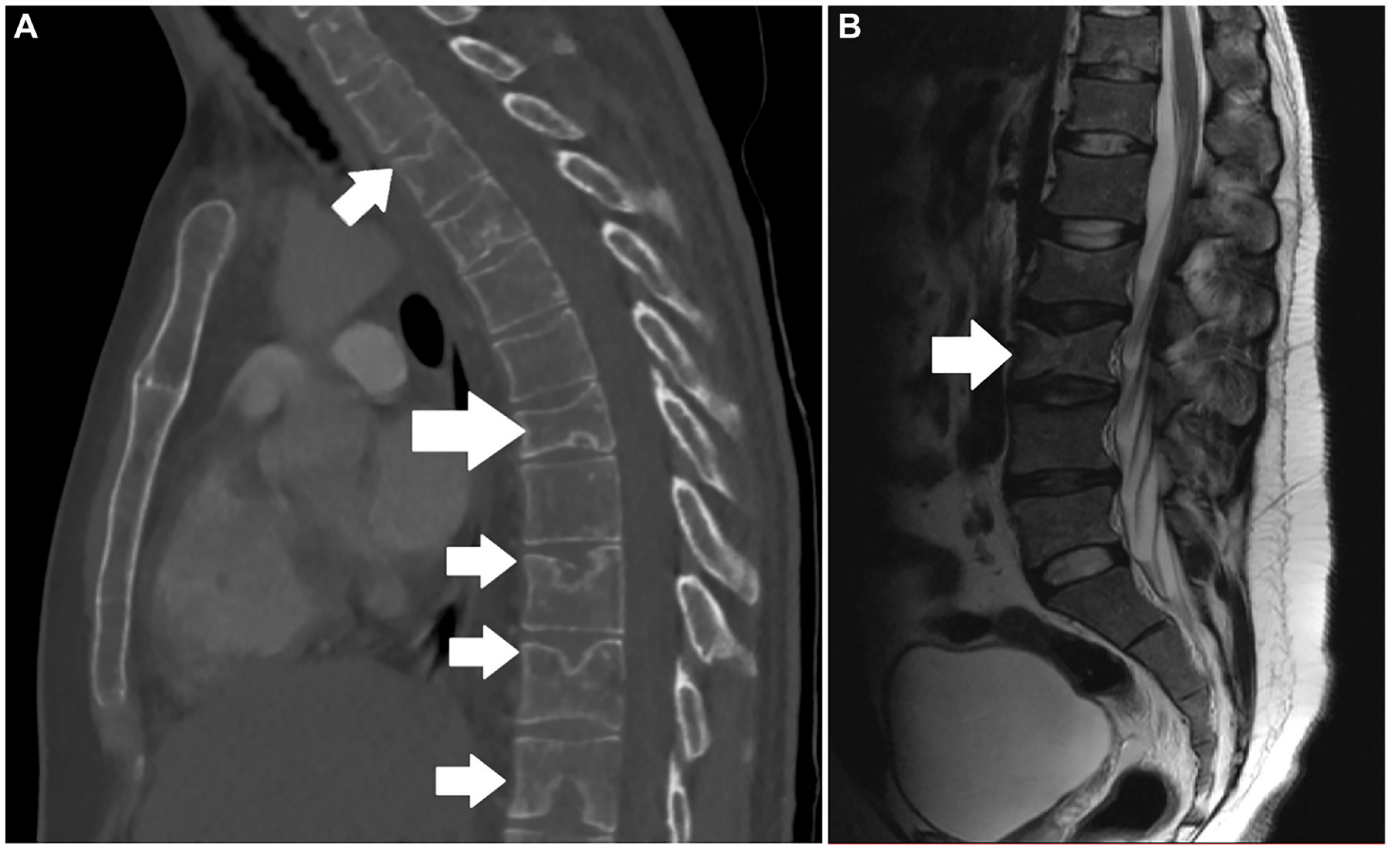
(A) Sagittal CT scan of the chest demonstrating a compression fracture in the T7 vertebra (large white arrow), along with osteoporotic changes in the epiphyseal plates of multiple vertebrae (smaller white arrows). (B) Sagittal MRI scan of the lumbar spine revealing a compression fracture in L3 (white arrow).
